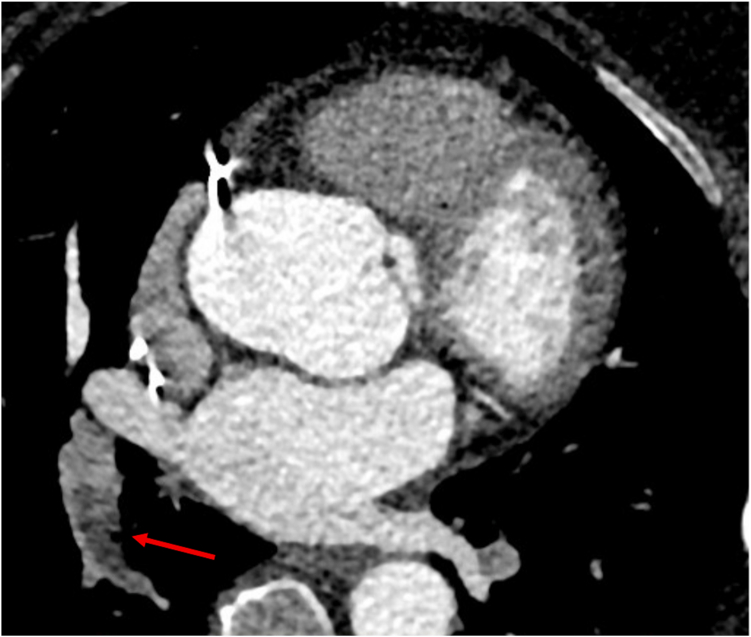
Computed tomography pulmonary angiogram demonstrating a partially occlusive filling defect (red arrow) within the distal right intralobar artery, extending into a medial segmental branch of right lower lobe artery.
